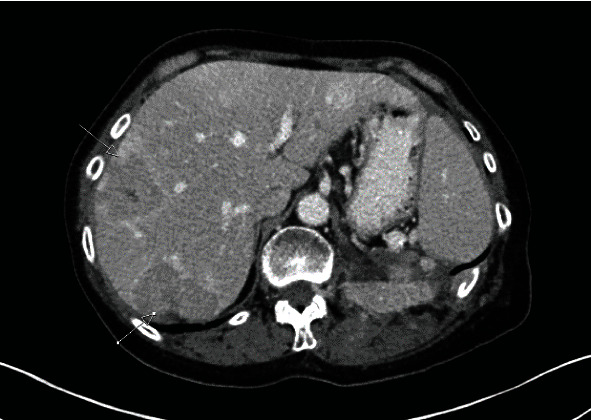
Follow-up CT of the abdomen showing liver deposits.
